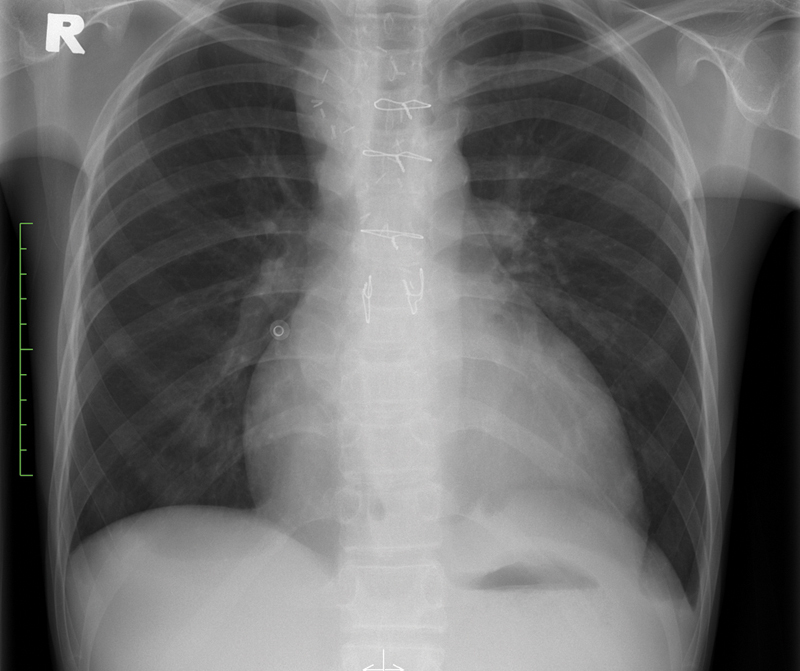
Postoperative frontal chest radiograph on outpatient follow-up was normal.
